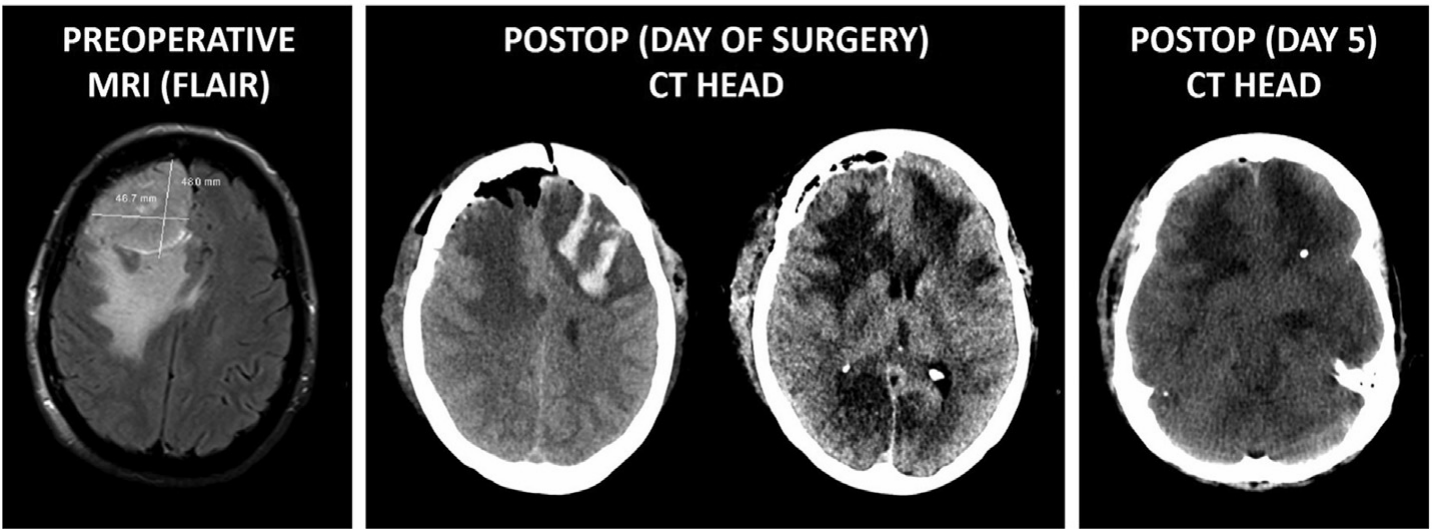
(a) Preoperative MRI: 5 cm right frontal meningioma with associated oedema. (b) Postoperative CT (Day 0): unexpected left frontal haemorrhagic infarct likely to represent venous infarction and new posterior circulation bilateral hypodensities. (c) Postoperative CT (Day 5): evolution of multifocal infarcts with obliterated basal cisterns and sulci, and uncal herniation.
